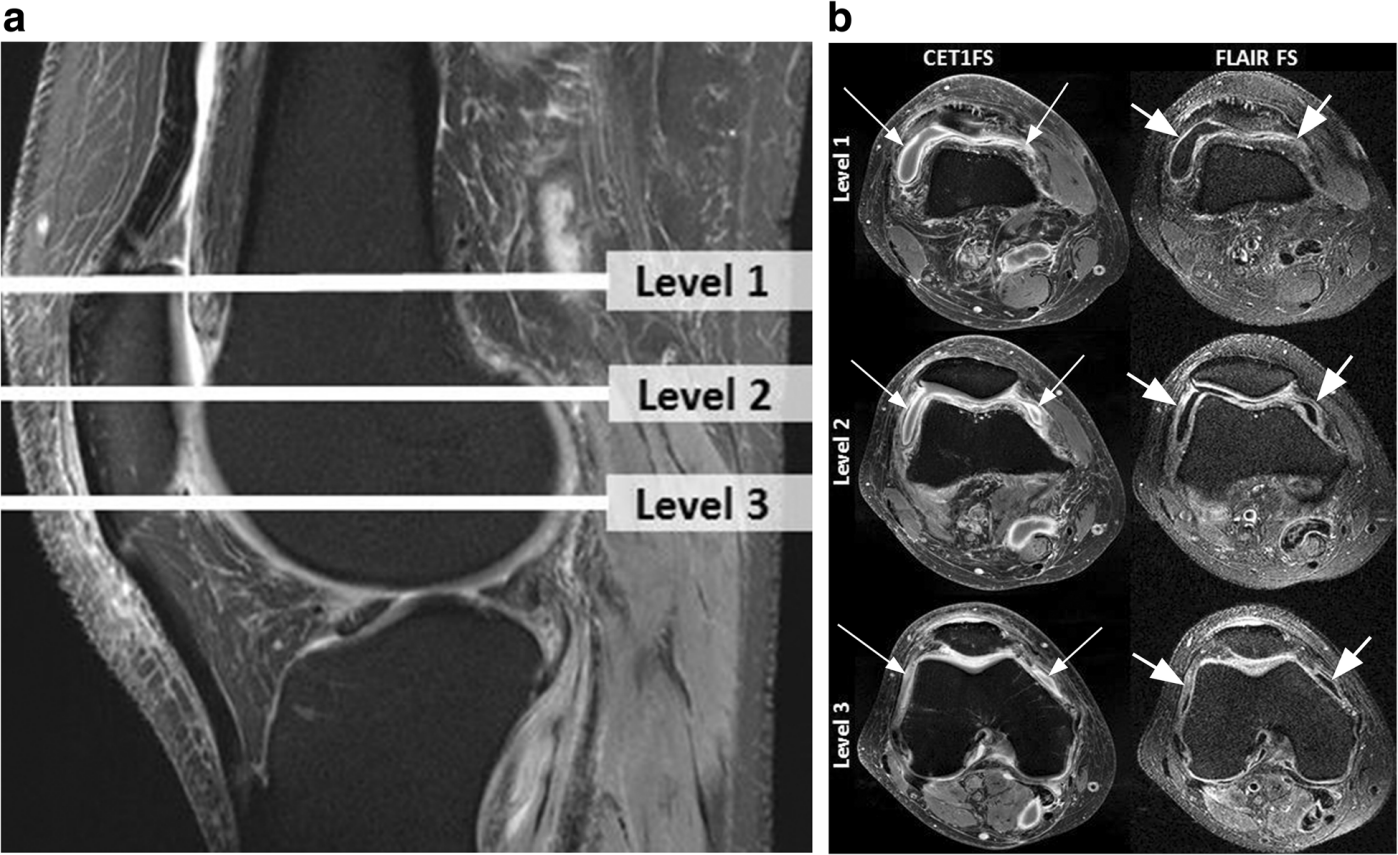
Anatomic coverage and visualization of synovitis on contrast-enhanced and non-enhanced sequences. a Sagittal intermediate-weighted fat suppressed (IW-FS) image shows the different axial levels that are depicted in b Level 1 represents the transverse slice at the level of the superior patellar pole. Level 2 is defined by the mid-point of the patella in the cranio-caudal direction and Level 3 is representing the transverse slice at the level of the inferior patellar pole. b Corresponding transverse image pairs of contrast-enhanced T1-weighted fat suppressed (T1-FS CE) and non-enhanced fluid attenuated inversion recovery fat suppressed (FLAIR-FS) sequences at each of the three levels of the femoro-patellar joint. Left figure column depicts the T1-weighted enhanced images with synovial thickening and contrast-enhancement at all levels (long arrows). Figure parts in the right figure column show corresponding FLAIR-FS images with synovitis being depicted in similar fashion as hyperintense with corresponding thickening of the synovial tissue at all levels (short arrows). Level 2 was used for semiquantitative assessment of peripatellar synovitis according to reference 16. Note that FLAIR images show synovial thickening to a somewhat lesser extent compared to T1-FS CE images
